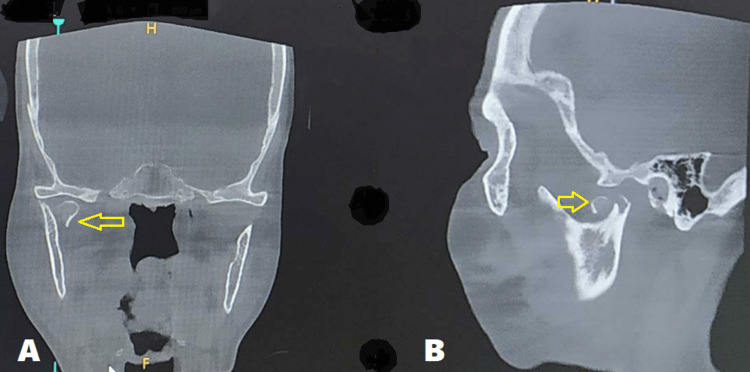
CT scan images CT scan showing medially displaced right condylar head fracture (arrows) in A) Coronal section B) Sagittal section
